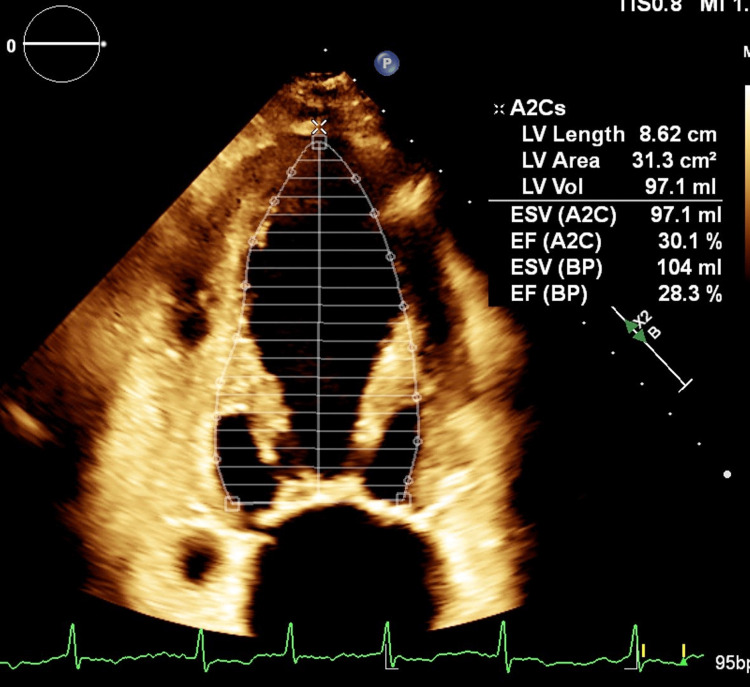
Transthoracic echocardiography showing global hypokinesis with severely reduced LVEF (25-30%), consistent with dilated cardiomyopathy LVEF, left ventricular ejection fraction
